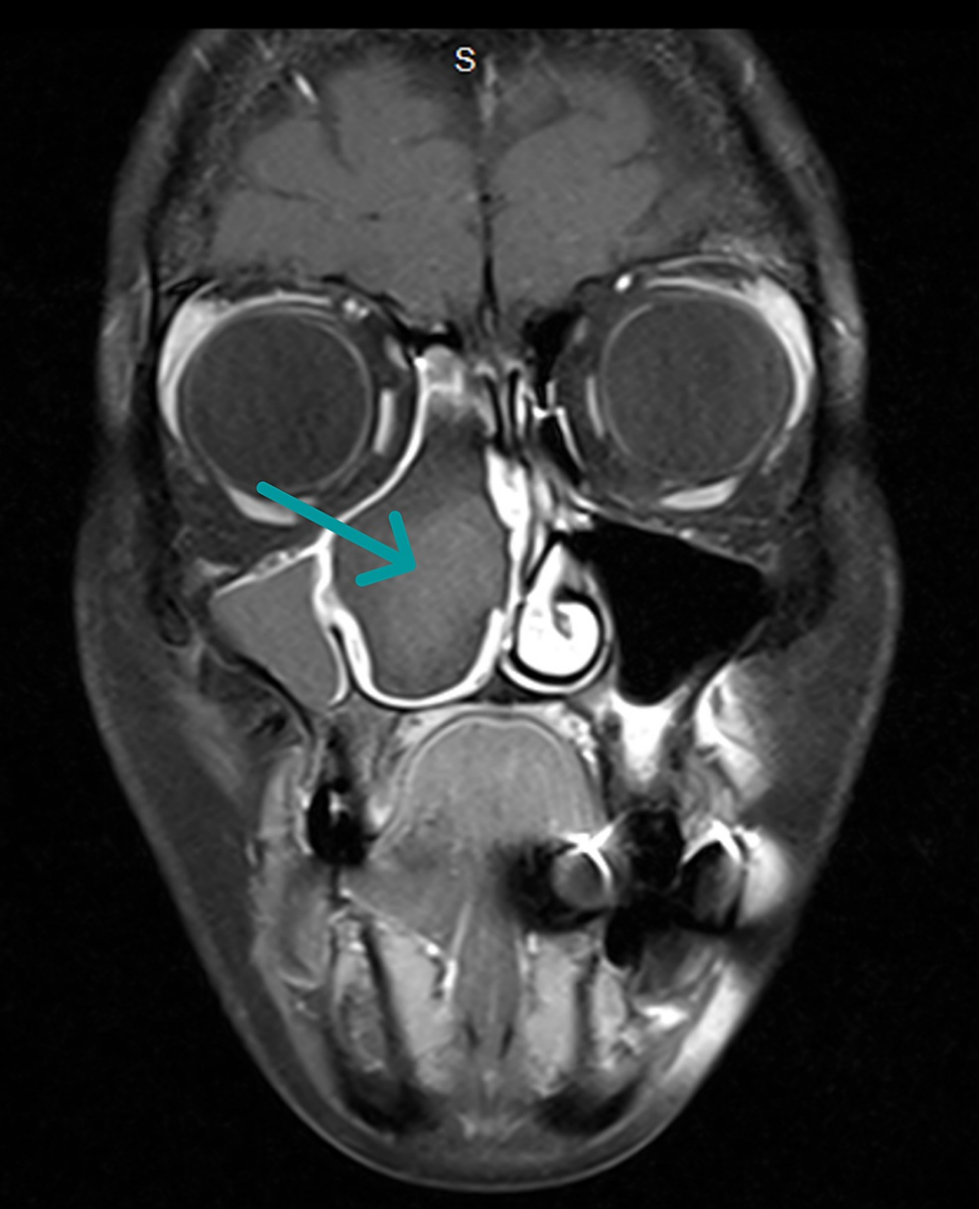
Brain and neck MRI The T1 coronal image shows a right maxillary sinus mass with iso-to-high signal intensity.
